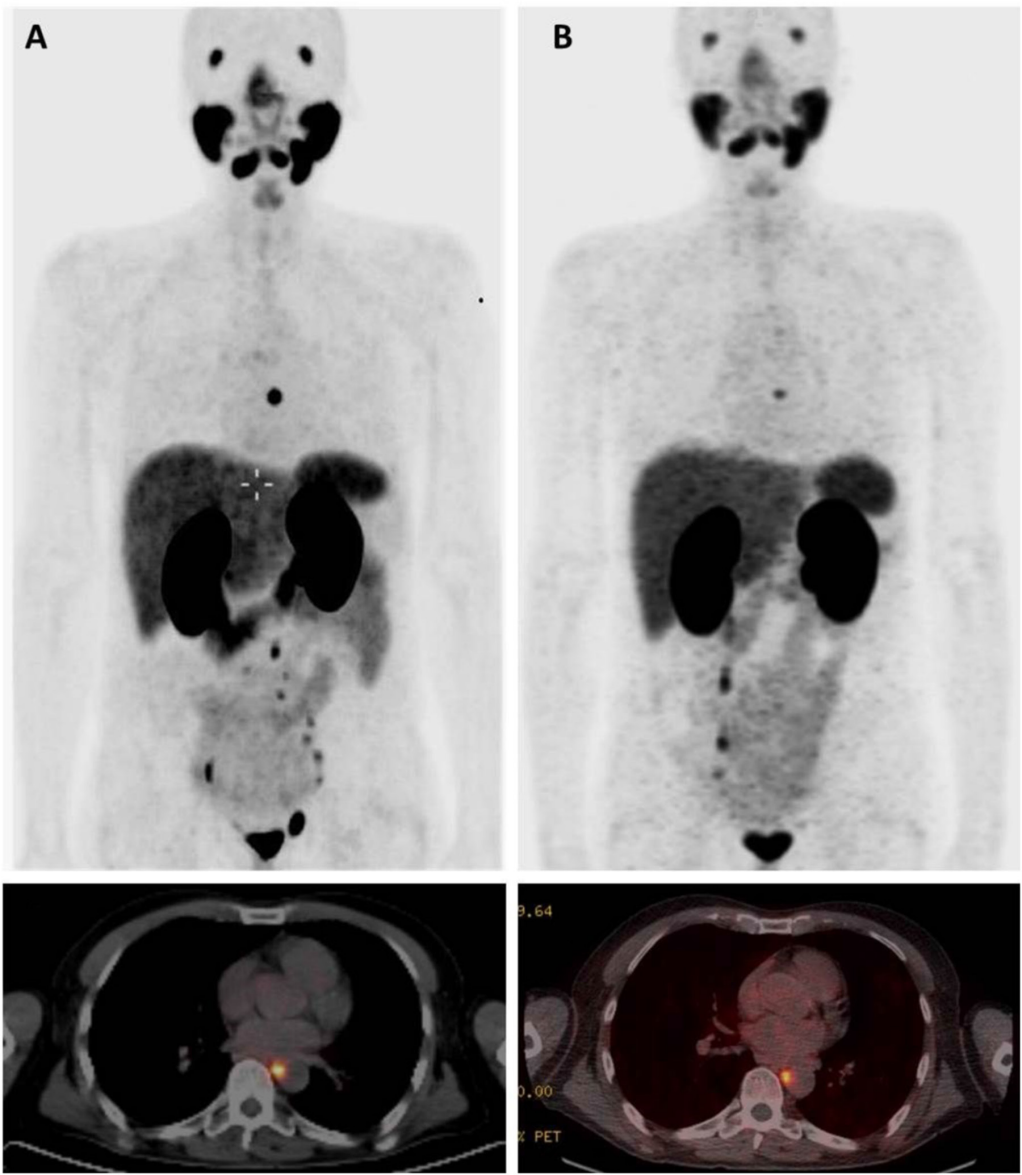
^68^Ga-PSMA-HBED-CC PET/CT before and after treatment with ^177^Lu-PSMA-617 RLT Panel A shows PET/CT before treatment July 2016 with the strongest lymph node uptake in a paraesophageal lymph node and uptakes in lymph nodes in the obturatory regions and sites in left internal and common iliac nodes. Panel B shows PET/CT after treatment November 2016 with uptake only in the mediastinal lymph node.
